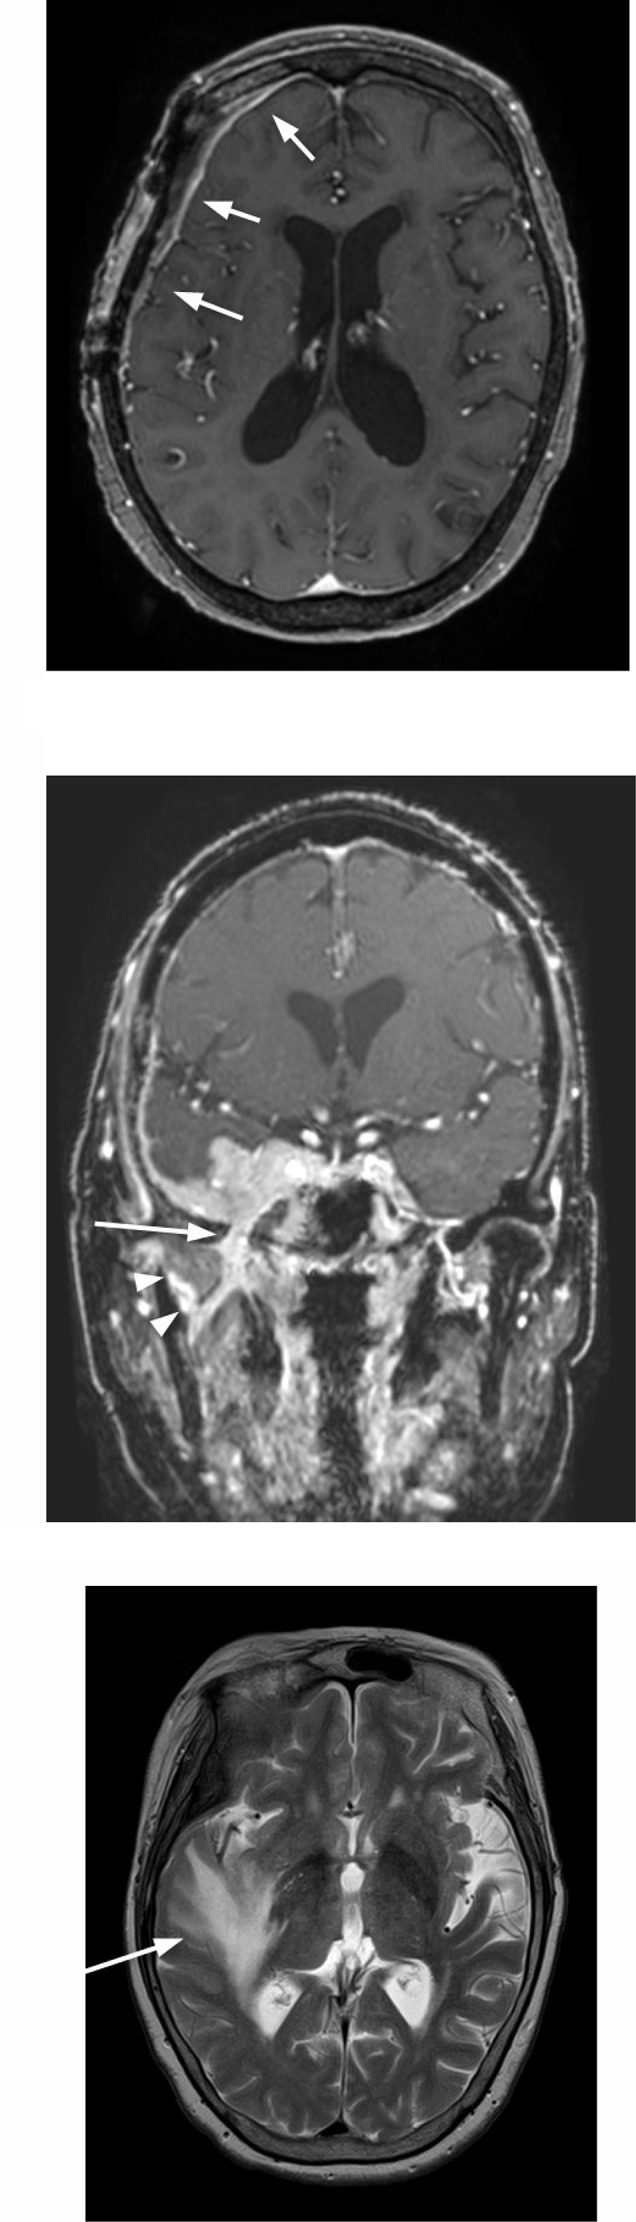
On contrast-enhanced, fat-suppressed, and T1-weighted images, dural thickening and enhancement (arrows)are also observed (A). Extracranial development via foramen ovale (arrows) and infiltration into pterygoid muscle (arrowheads) are demonstrated (B). On the T2-weighted image, cerebral edema in the right frontotemporal robe is remarkable (arrows, C).
